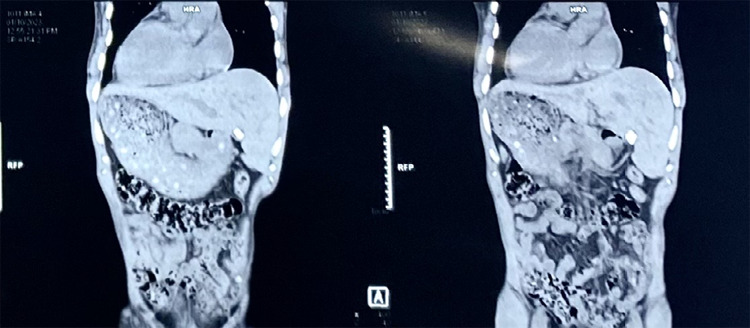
CT scan showing situs inversus totalis.
